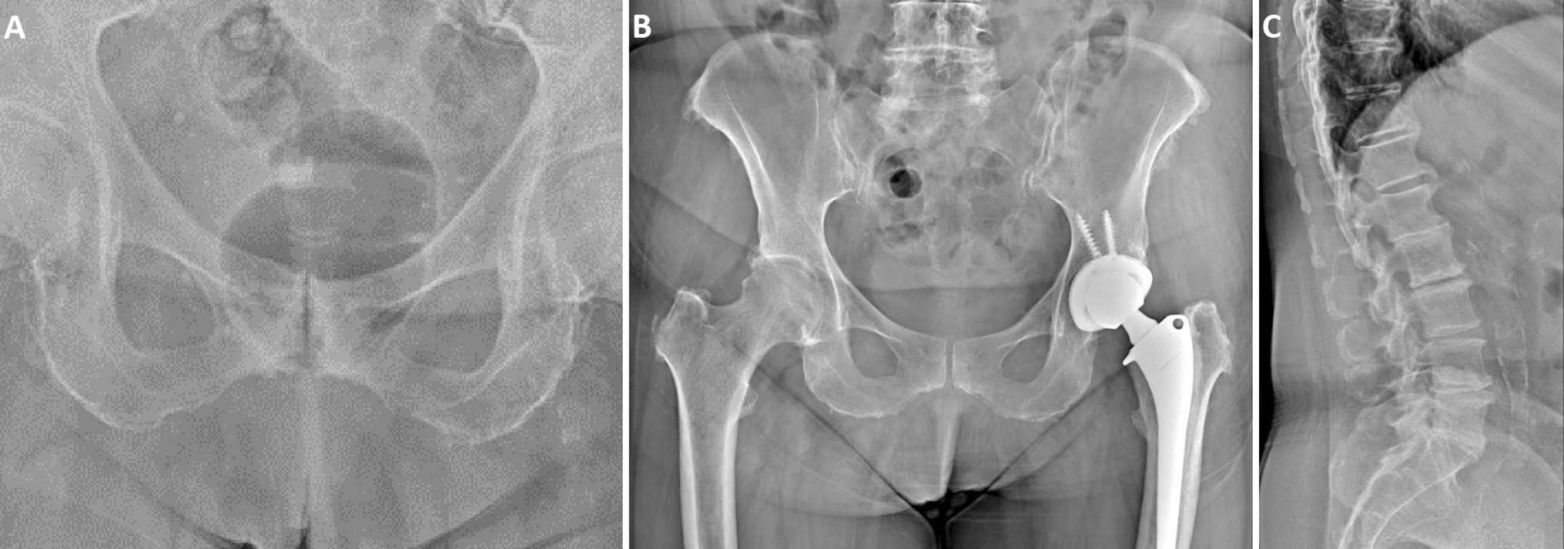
Osteitis pubis, pelvic enthesopathies and aortic calcification. a Anterior–posterior radiograph of the pelvis showing osteitis pubis with sclerosis and erosions of the pubic symphysis; b anterior–posterior radiograph of the pelvis showing bilateral enthesopathy of the iliac crest, ischiopubic ramus and greater trochanter. c Lateral radiograph showing flowing bridges involving the thoracolumbar spine, ossification of the interspinous ligament and calcification of the abdominal aortic wall
